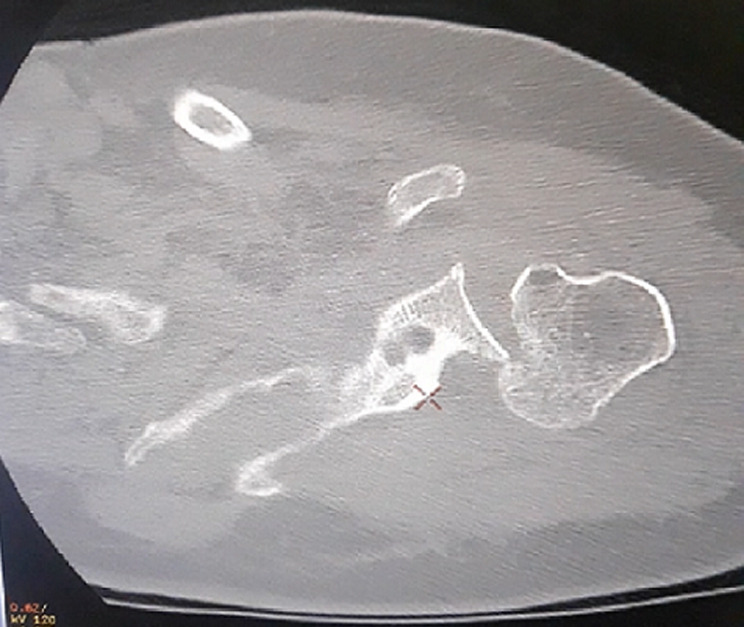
CT image showing a reverse Hill-Sachs lesion
